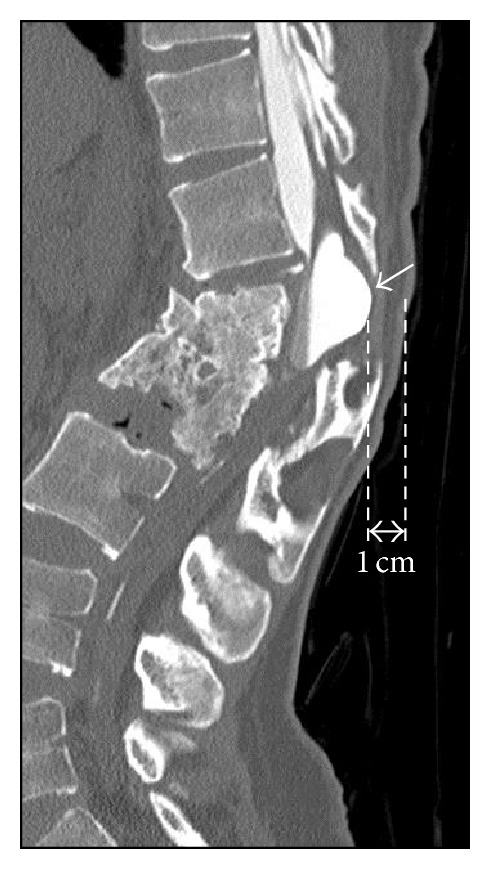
Computed tomography myelography (CTM) revealed a pouch-like total blockage at the L1 level (short arrow) and a 1 cm distance between the body surface and the dura mater with atrophied back muscles.
